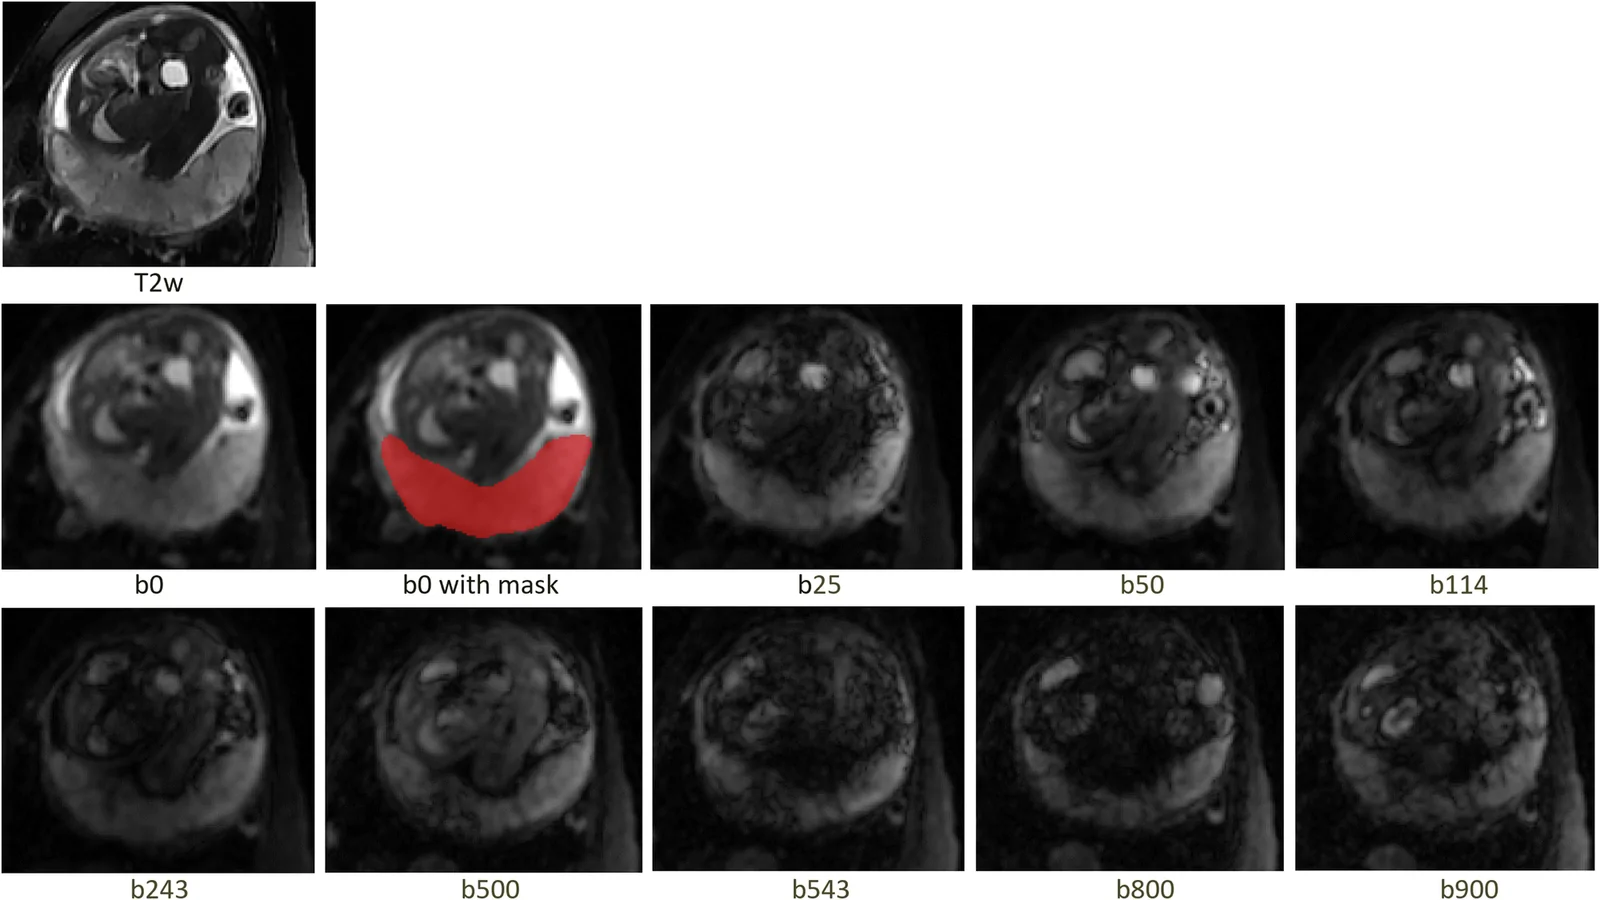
Placenta imaging. Placenta T2-weighted MRI scan (1st row) and diffusion weighted imaging scans with different b values (2nd and 3rd rows) of a subject at 30.29 gestational weeks.
